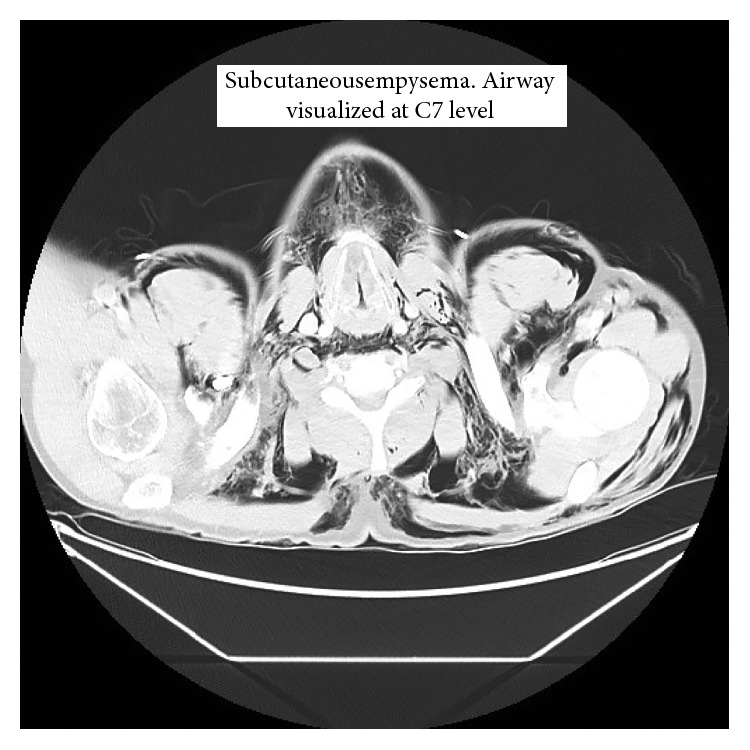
Axial CT image at the level of the thyroid cartilage demonstrating air infiltration around the larynx. There is notable swelling of the soft tissue of the larynx.
